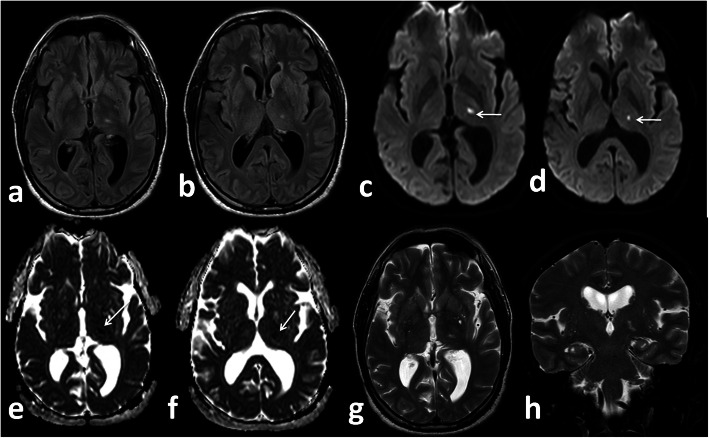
Male patient 48 years old presented with sensory loss. Left thalamic infarction. DWI (white arrow in c and d), ADC map (white arrows in e and f)
